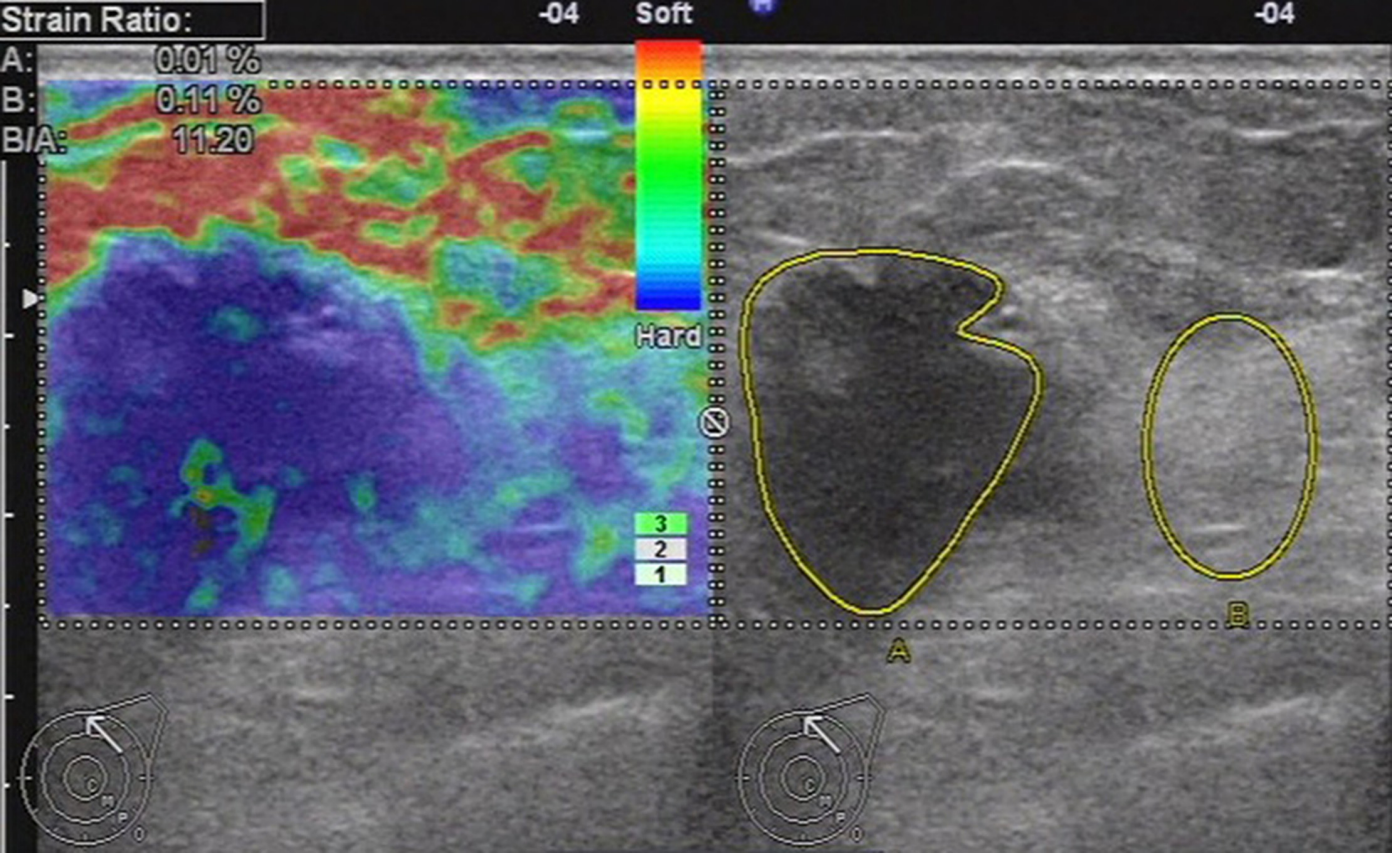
Ultrasonic elastographic image of a IDC in a 56-year-old woman. The lesion was scored 5 with the 5-point scoring method. The strain ratio was 11.20 with the strain ratio method at the same breast tissue depth as the reference.
